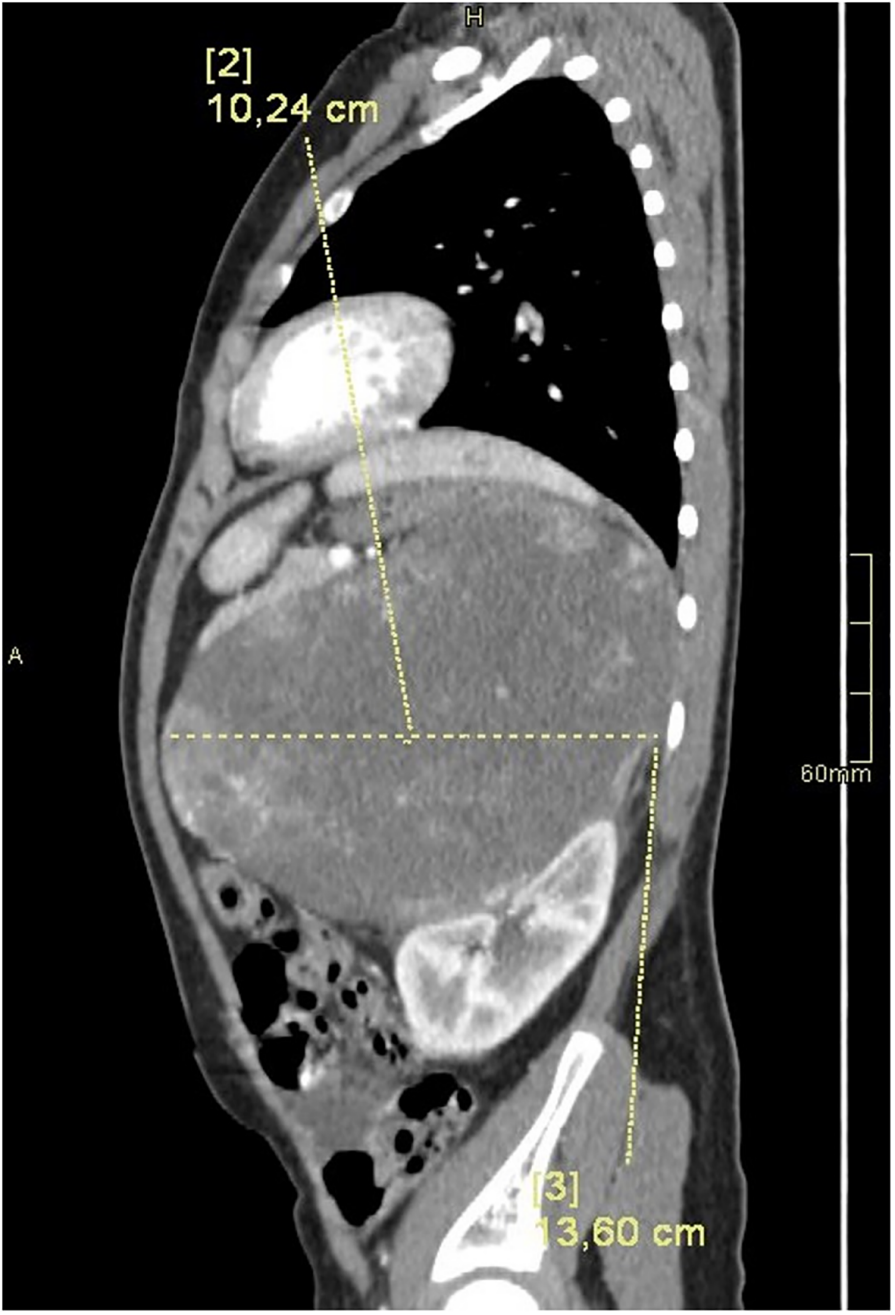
CT of the abdomen in the LR plane.
